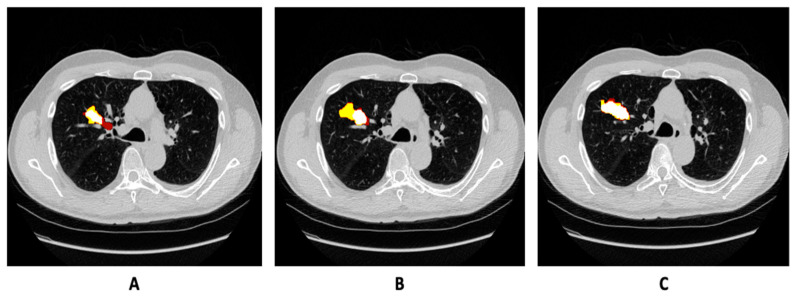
(A–C) Normalized image slices of CT scan of same patient with growing tumor in MSD-2018 training set, along with annotation overlaid on the image.
